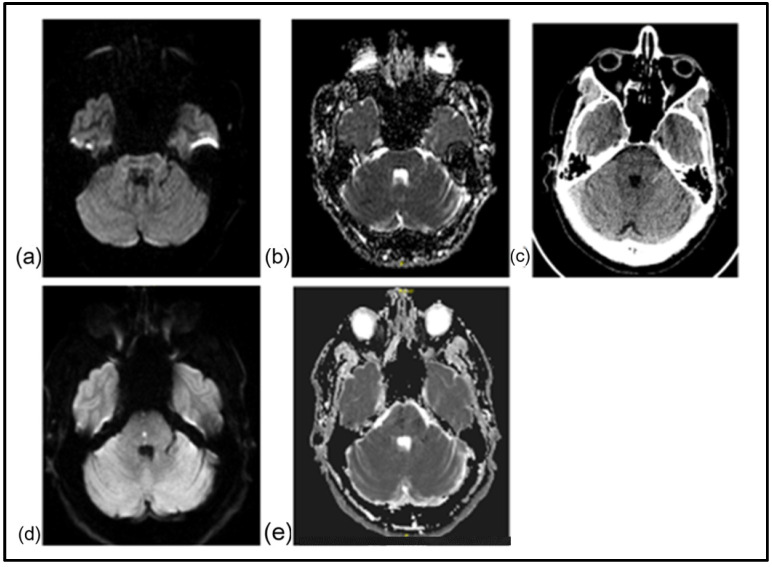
A 49-year-old man presented with dizziness. Clinical examination revealed isolated internuclear opthalmoplegia, indicative of a dorsal pontine lesion. In the initial scan 2 h after symptom initiation, the DWI (a) and ADC maps (b) were normal. No signs of bleeding were noted in NCCT (c) and the patient received intravenous thrombolysis with tenecteplase. Repeat imaging within 24 h revealed a small DWI hyperintensity at the level of the pons (d), along with a corresponding decrease in ADC map (e) consistent with infarction in the area of the medial longitudinal fasciculus. The patient’s symptoms had improved at discharge (NIHSS score: 1, modified Rankin score: 1) and an excellent outcome with no disability (NIHSS score: 0, mRS: 0) was documented at follow-up 3 months later.
